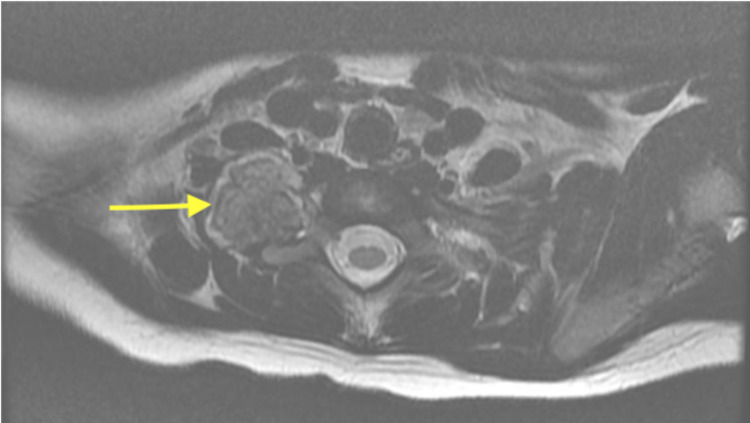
MRI of cervical spine (axial T2) revealing osteochondroma of the right first rib (at yellow arrowhead) MRI - Magnetic Resonance Imaging Right paraspinal mass that appears to arise from the right first rib with continuity of the medullary cavity and bright peripheral T2 signal suggesting a cartilage cap. Findings are most consistent with osteochondroma. However, given the widening of the adjacent neural foramina and apparent extension to the neural foramina, ganglioneuroblastoma could be considered, though this would be less likely given the profuse calcification.
